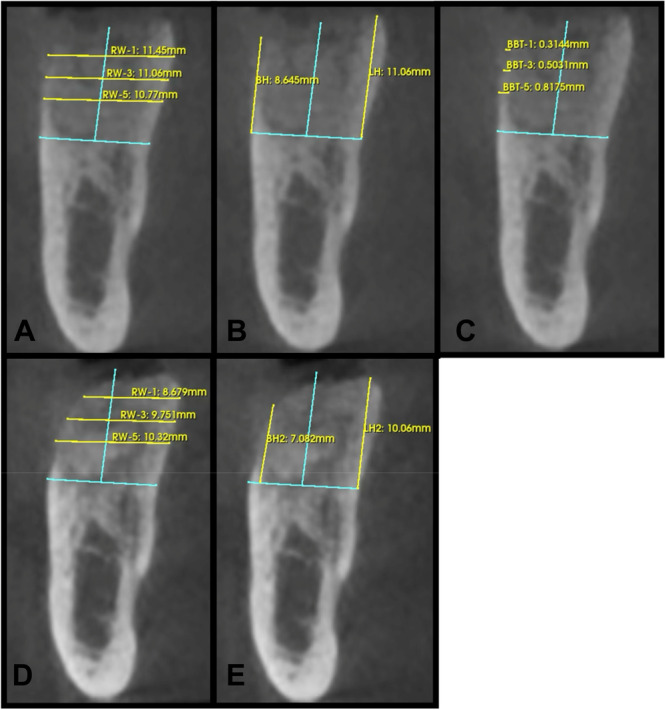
A clinical case of ridge preservation at site #30 demonstrating radiographic measurements. (A) Baseline measurements of ridge width at 1 mm (RW‐1), 3 mm (RW‐3), and 5 mm (RW‐5) apical to the crest. (B) Baseline measurements of Buccal height (BH) and Lingual Height (LH). (C) Baseline buccal bone thickness at 1 mm (BBT‐1), 3 mm (BBT‐3), and 5 mm (BBT‐5) apical to the crest. (D) 3‐month postoperative ridge width measurements at all levels and (E) 3‐month postoperative buccal and lingual height measurements. All measurements were taken with the same vertical and horizontal reference lines (in blue) at both time intervals.
